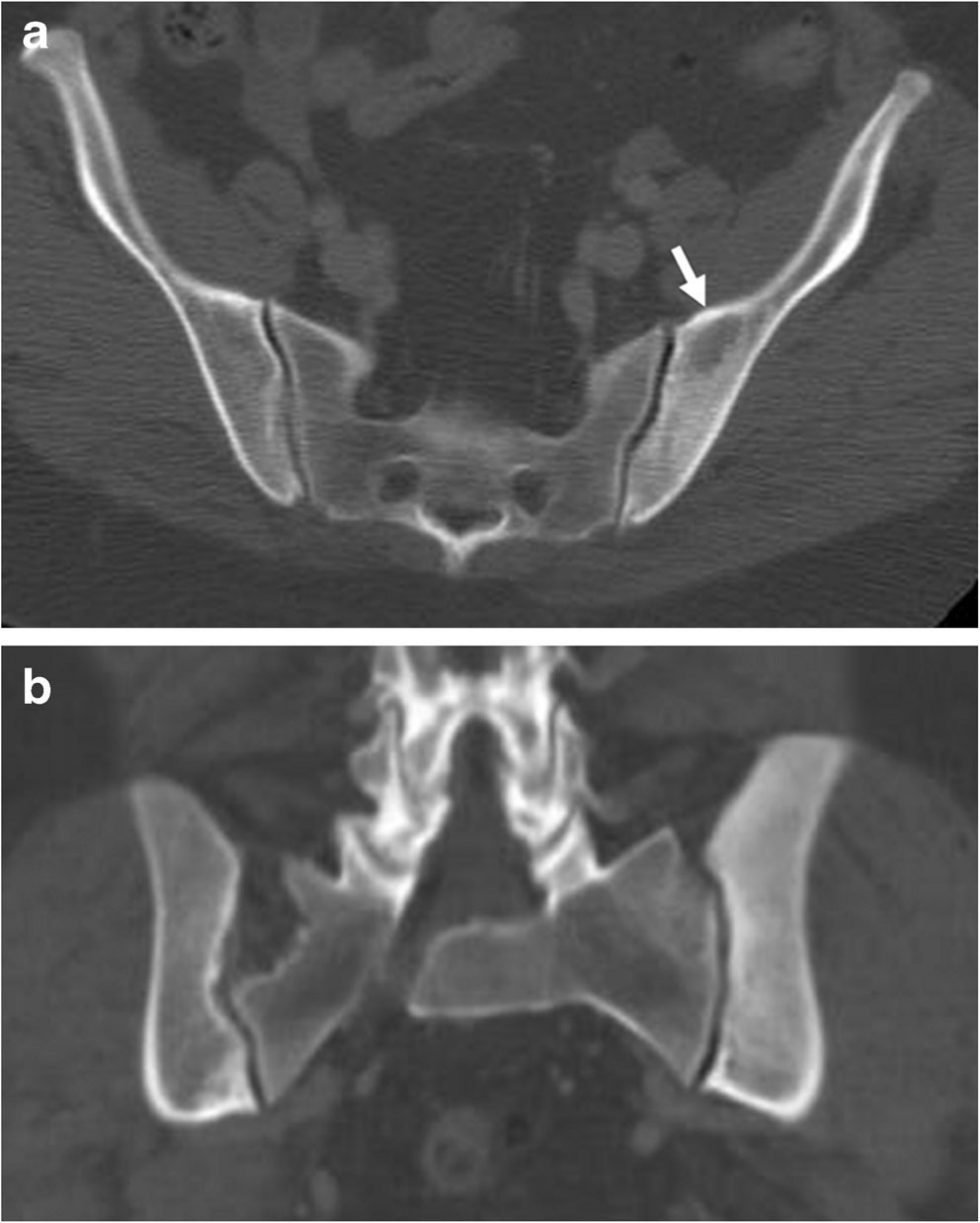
a, b: Unenhanced CT. Sacral region, axial plane (a) and coronal reformation (b). Renal osteodystrophy of the left iliac wing with diffuse bone sclerosis and some areas of resorption (*arrows*) due to secondary hyperparathyroidism in a patient undergoing chronic haemodialysis
